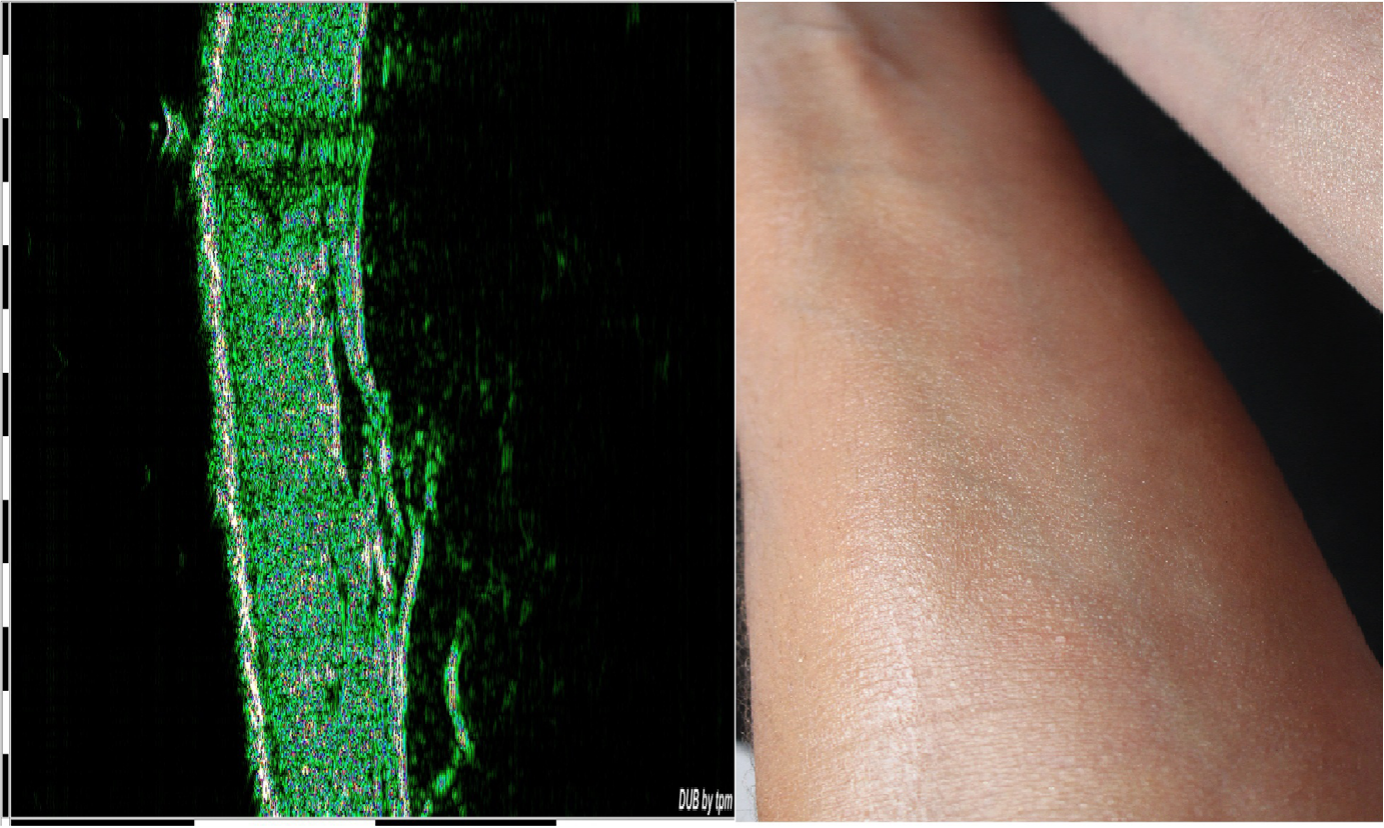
Sample of healthy skin and its sonogram.
